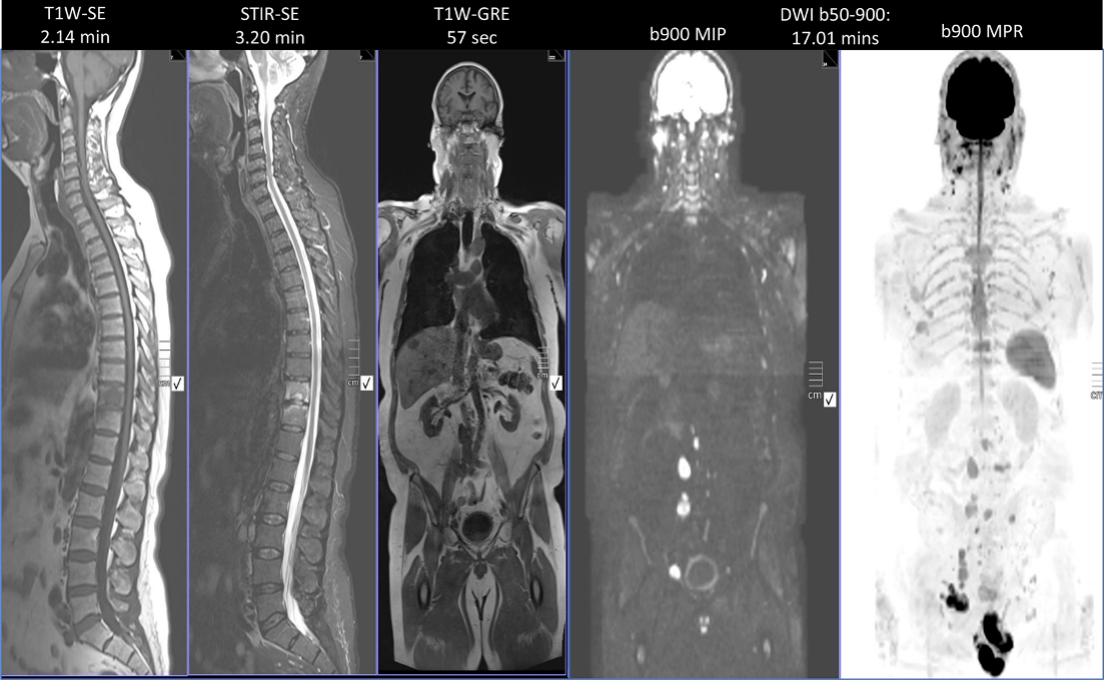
Standard protocols for whole-body magnetic resonance imaging (MRI) for bone metastasis include a short MRI protocol in <30 min (detection) and a comprehensive MRI protocol in 45 min (response). (Courtesy of Prof. Anwar Padhani, © Prof. Padhani. This content is not part of the Open Access License.)
